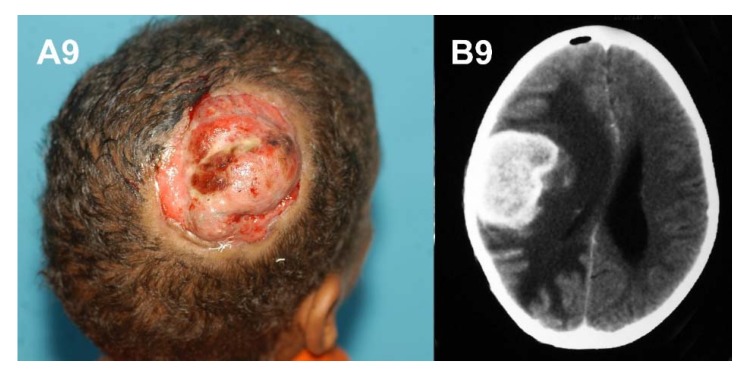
(A) massive head eumycetoma. (B) CT scan showing intra-cranial extension.
